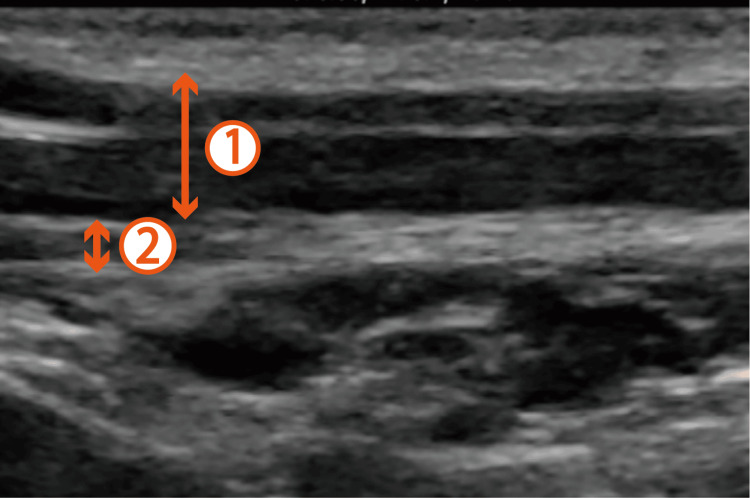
Ultrasound-guided selective glossopharyngeal nerve block in Case 2. The sternocleidomastoid muscle and the stylohyoid muscle can be observed behind the mandibular ramus. 1: Sternocleidomastoid muscle; 2: Stylohyoid muscle.
